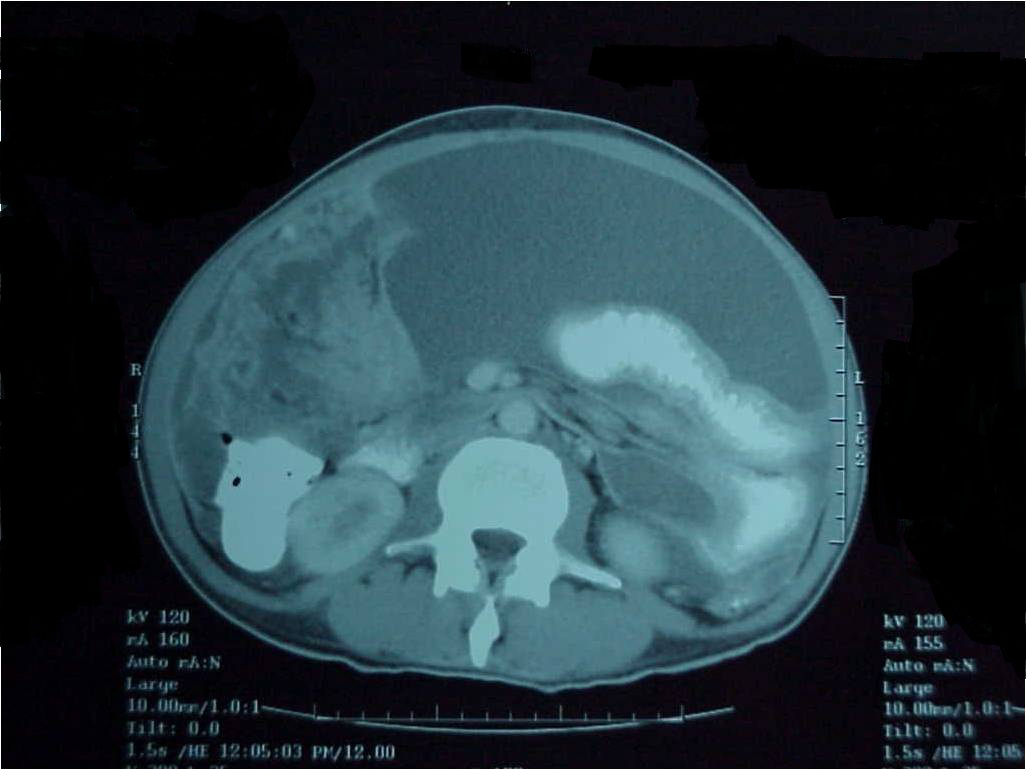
Computed tomography scan with intravenous contrast enhancement showing the thickened gut wall and mesentery with the presence of ascites.
